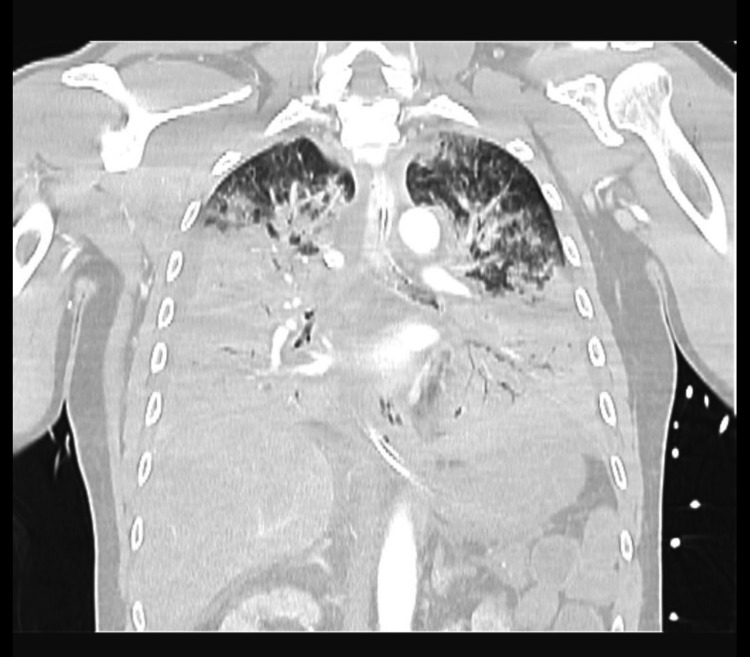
Thoracic-abdominopelvic CT on the second admission showing extensive lung consolidation bilaterally consistent with congestion
